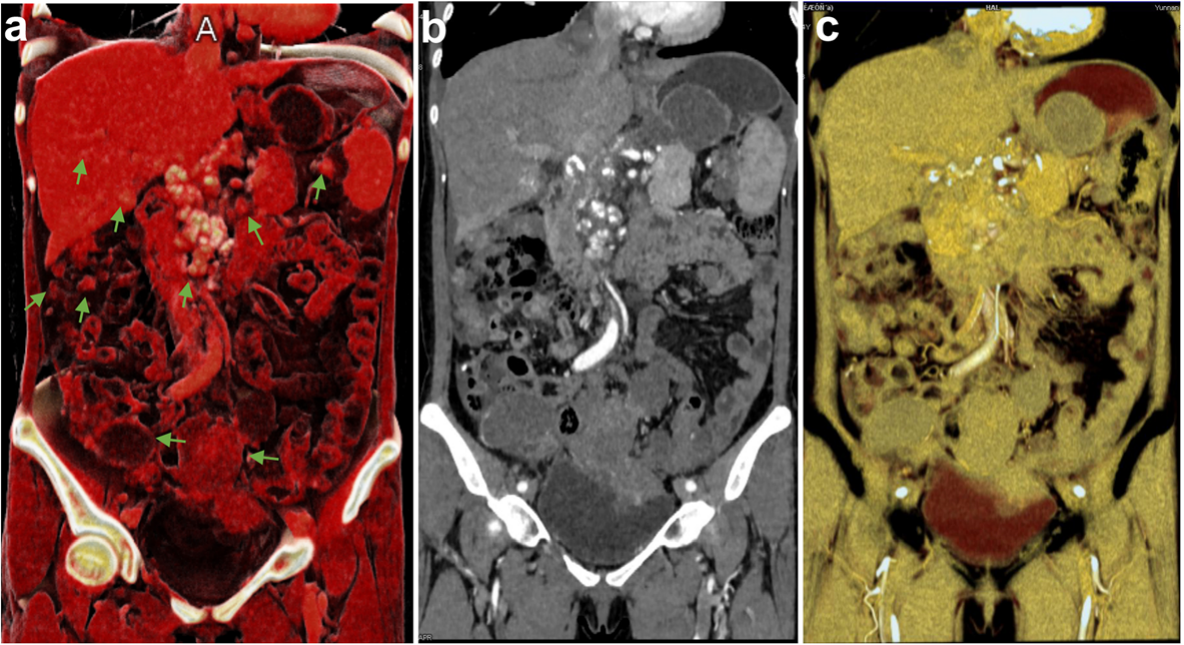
CT images of the abdomen and pelvic cavity in a patient with recurrent ovarian cancer and metastases (case 1). Recurrent nodules and masses in the pelvic cavity, multiple metastatic nodules in the right para colonic sulcus, splenic hilum and hepatic parenchyma, and metastatic lymph nodes in the hepatic portal area and the upper middle abdominal peritoneum. (a) CR image showed that the intra-abdominal metastatic nodules had strong stereo effects. The relationship between the lesions and peritoneum and colonic adhesions was clear. Metastatic lymph nodes showed a higher brightness of white because of calcification, while the soft-tissue components around the calcified lesions were also demonstrated clearly. (b) The MPR image showed the overall view of the lesions similar to that of CR, but the gray-level image was less detailed and lacked a better texture. The relationship between the metastatic lymph nodes and the adjacent duodenum and the abdominal vessels was not clear. (c) Because of the close density between the metastases and the gastrointestinal tract in the VR image, the lesions can hardly be distinguished. The display effect for other structures in the abdominal cavity was also poor
